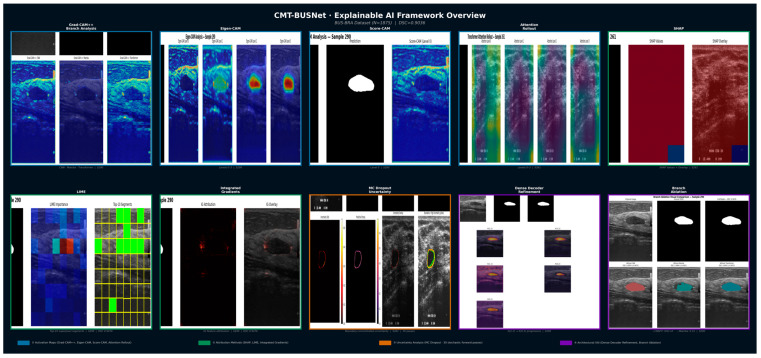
Explainable AI (XAI) Framework Overview of CMT-BUSNet Across Eleven Complementary Analysis Modules. Representative XAI visualizations applied to breast ultrasound samples from the BUS-BRA dataset. Top row: Grad-CAM++ branch-level activation maps (CNN, Mamba, Transformer; Sample 290, DSC = 0.9476), Eigen-CAM, Score-CAM, Attention Rollout, and SHAP pixel attributions (Sample 261, DSC = 0.8976). Bottom row: LIME superpixel importance, Integrated Gradients, MC Dropout uncertainty (30 stochastic forward passes; Sample 261), Dense Decoder progressive refinement X (2,1) X (0,3) (Sample 290), and branch ablation comparison (Sample 290). Color-coded borders indicate analytical group: blue = activation maps, green = attribution methods, orange = uncertainty analysis, purple = architectural XAI. Key observation: Attribution methods (SHAP, LIME, Integrated Gradients) converge on the hypoechoic tumor region through independent methodologies, while activation-based methods highlight perilesional context, confirming multi-perspective interpretability.
